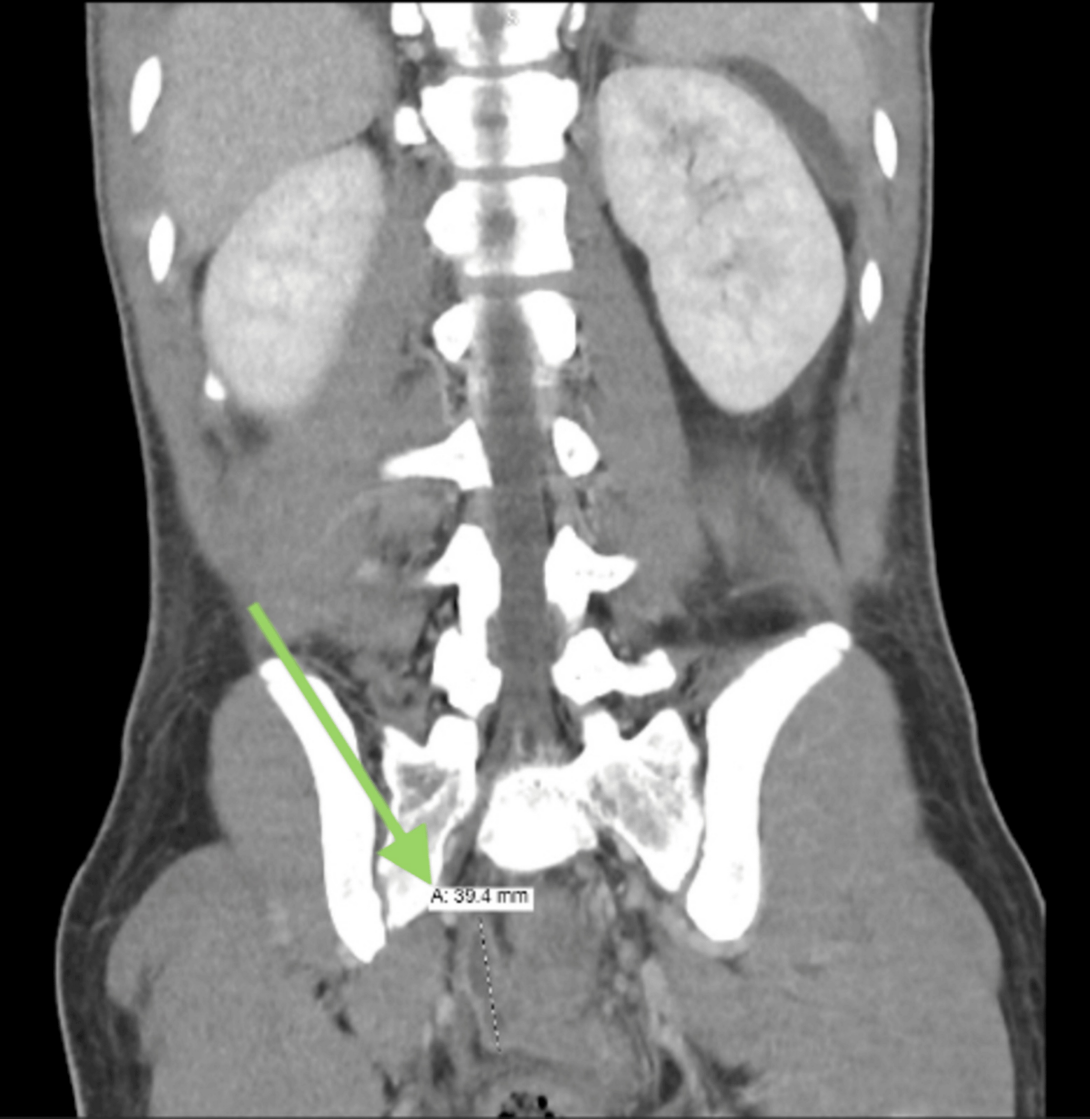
Follow-up CT image displaying progressive thickening and enhancement of the peritoneum with increased ascitic fluid, consistent with ongoing peritonitis.
